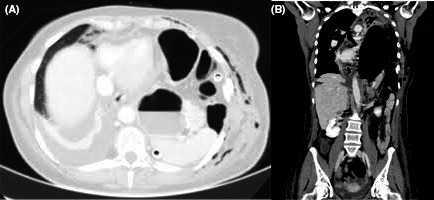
A,B, CT view of intrathoracic intestinal herniation
